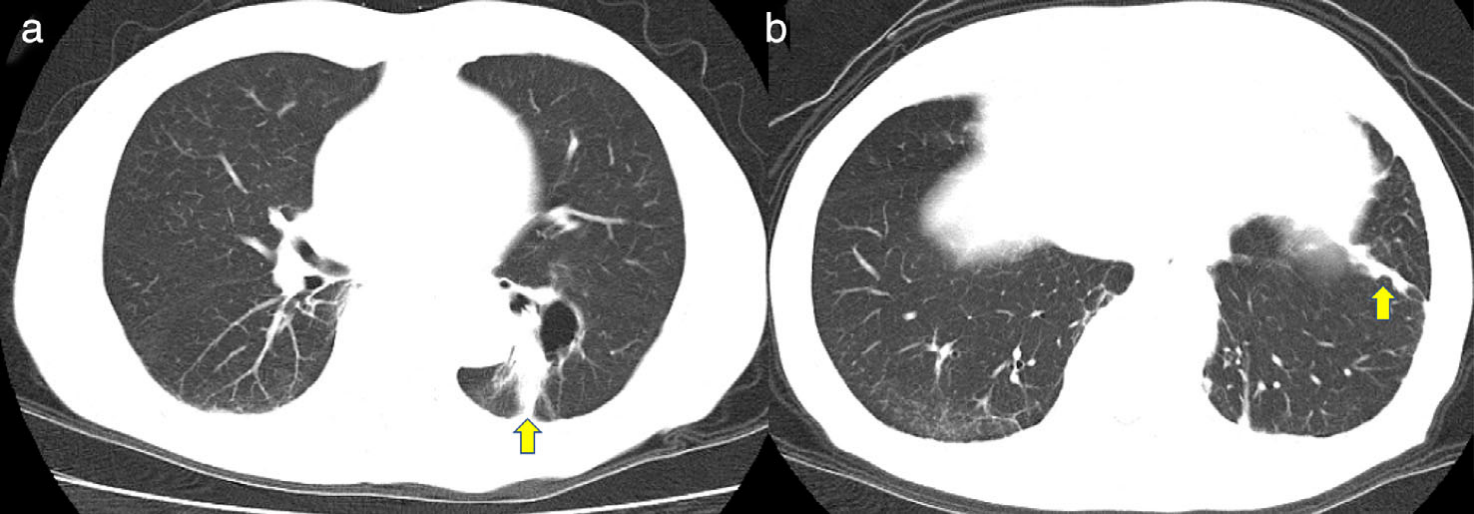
Chest computed tomography (CT) findings before the start of afatinib treatment. The yellow arrows indicate the tumor adjacent to the cyst (a) and pleural dissemination (b)
